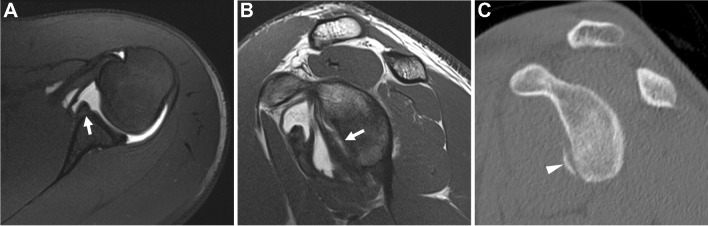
Anterior instability. Twenty-year-old man with recent anterior glenohumeral dislocation while playing rugby. Axial T1-weighted fat-suppressed (A) and sagittal T1-weighted (B) 1.5 T MR arthrogram images demonstrate a mixed fibrocartilaginous and osseous Bankart variant lesion with deficiency of the anterior glenoid (arrows). C Sagittal reformat image from CT scan 4 months later demonstrates the displaced osseous Bankart component (arrowhead)
